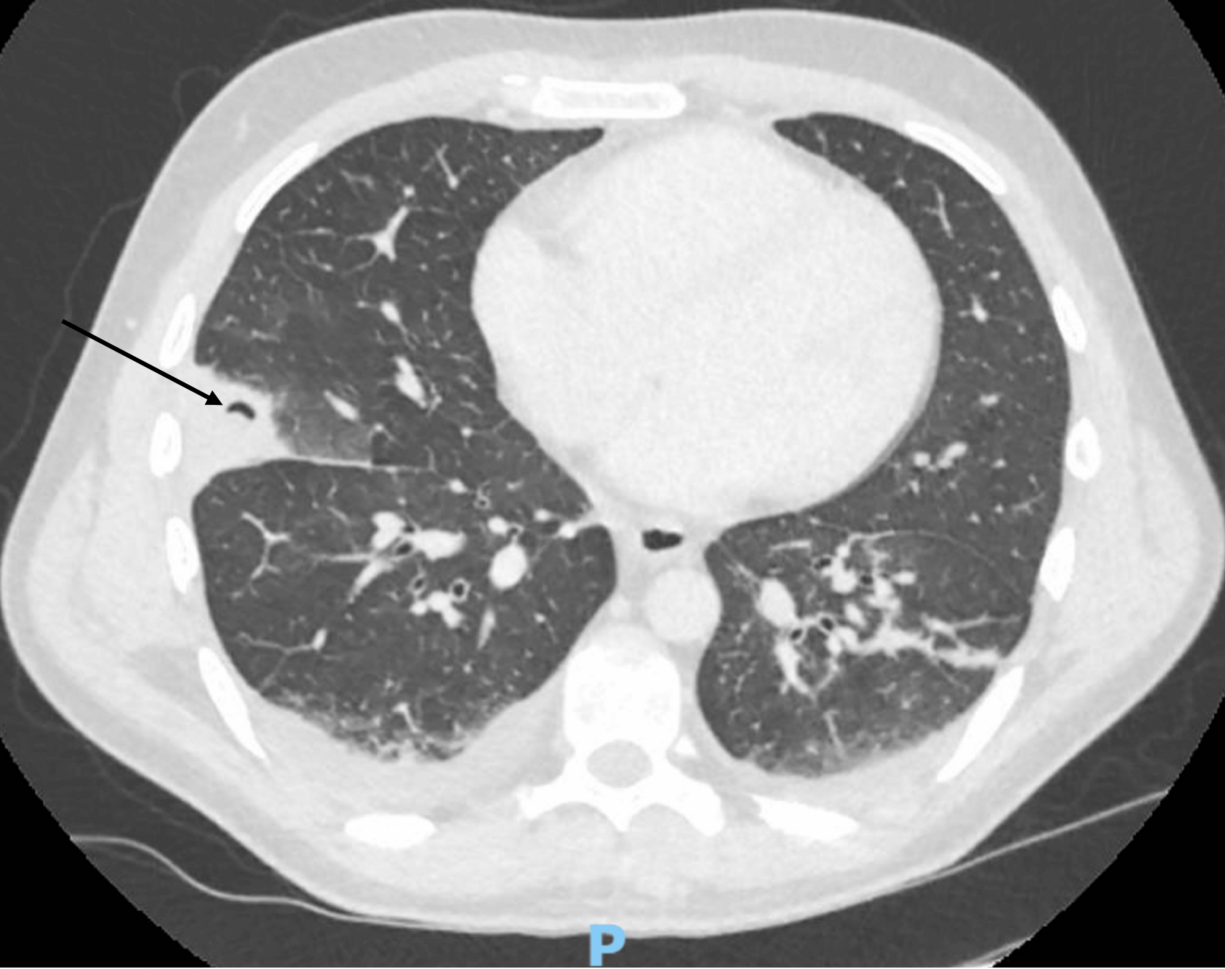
CT of the chest showing a right-sided wedge-shaped consolidation with surrounding cavitation (arrow)
